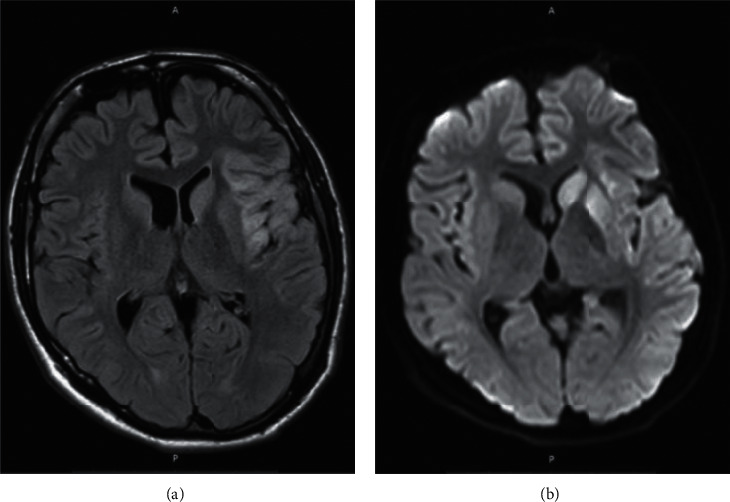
Brian MRI images of the patient, two weeks after neurological symptom onset. Axial (a) fluid-attenuated inversion recovery (FLAIR) MRI scans and (b) diffusion-weighted images (DWI) showing a hyperintense signal and edema of the caudate nucleus head, putamen, and parts of the external capsule and insula on the left side.
